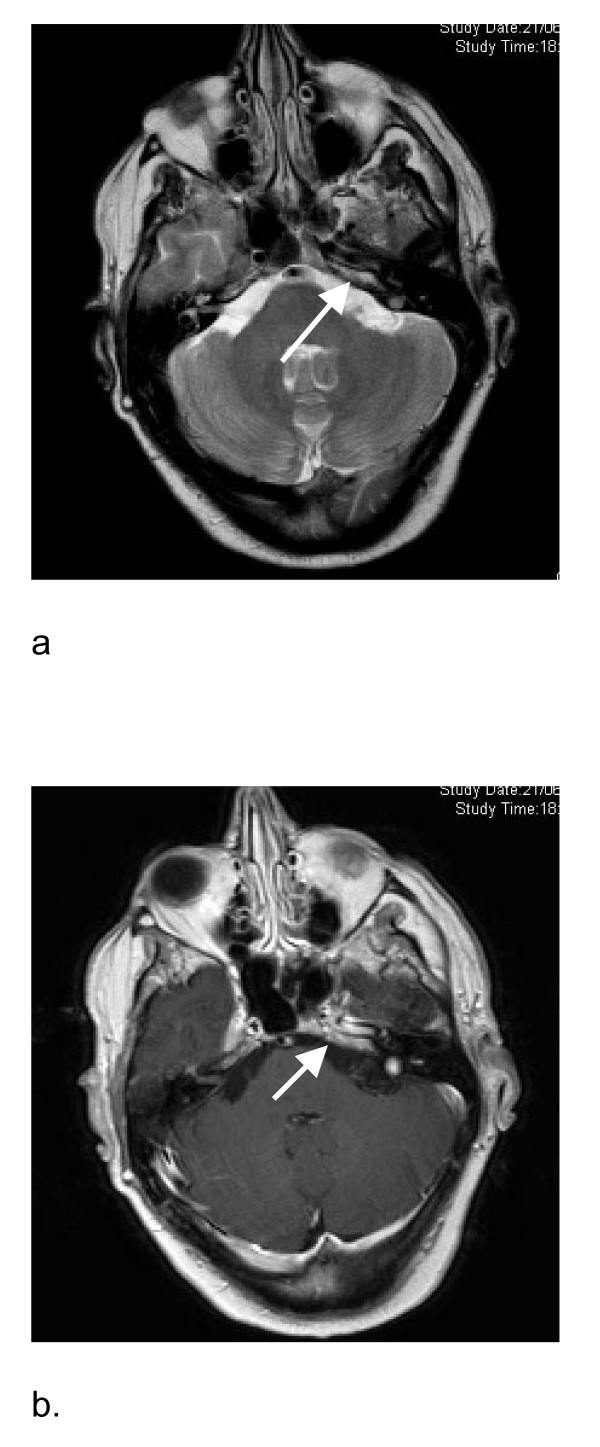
Brain magnetic resonance images. (a) Brain magnetic resonance image scanning T2 weighted image showing high signal of the fifth, sixth, seventh and eighth cranial nerves (arrow). (b) Brain magnetic resonance image scanning T1 after gadolinium showing enhancement of the fifth, sixth, seventh and eighth cranial nerves (arrow).
